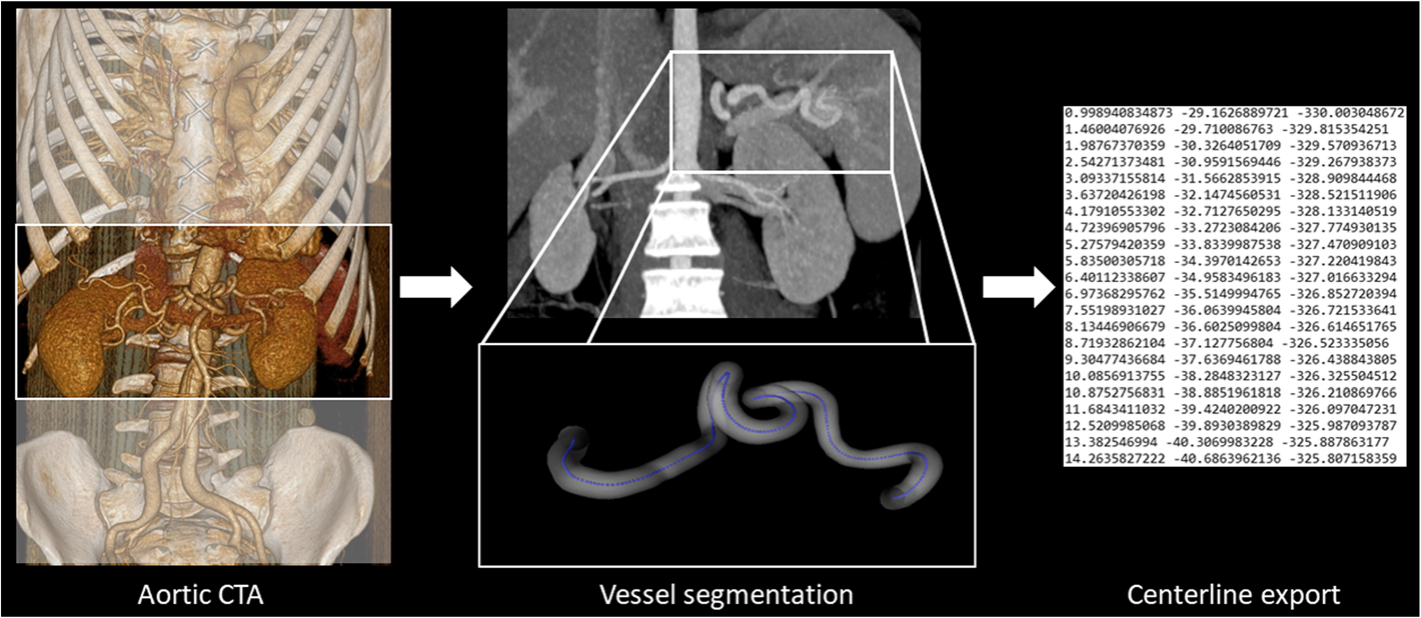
To measure tortuosity abdominal CT angiography images of patients with MFS and age and gender matched controls were selected. After manual segmentation of the vessels 3D coordinates of the centerlines were exported to calculate tortuosity metrics
